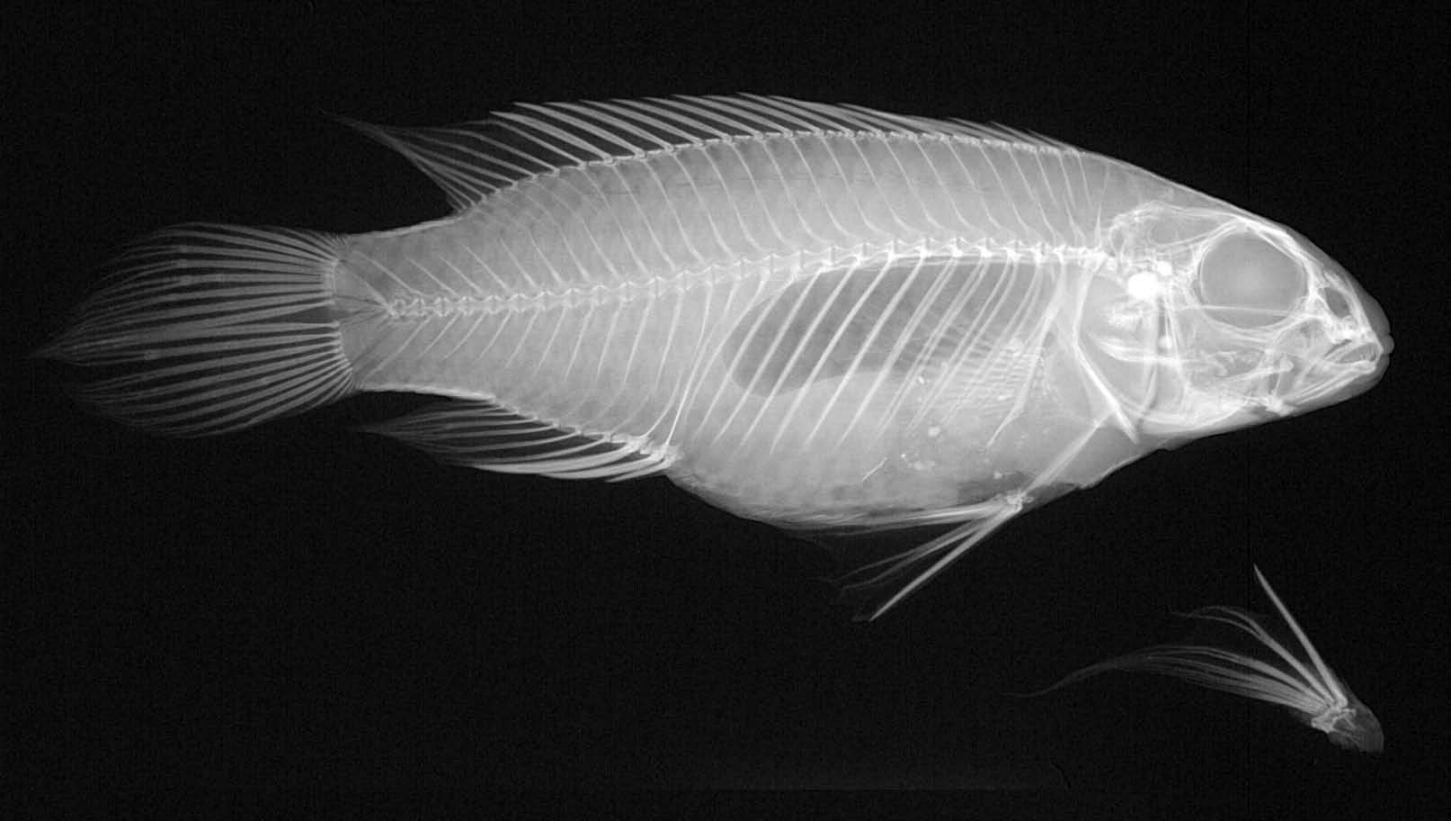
Sample of an X-ray image. Example of an X-Ray image of a female *P. taeniatus*. One of the paired pelvic fins was dissected in order to avoid errors in measurements by overlap in X-Rays.
